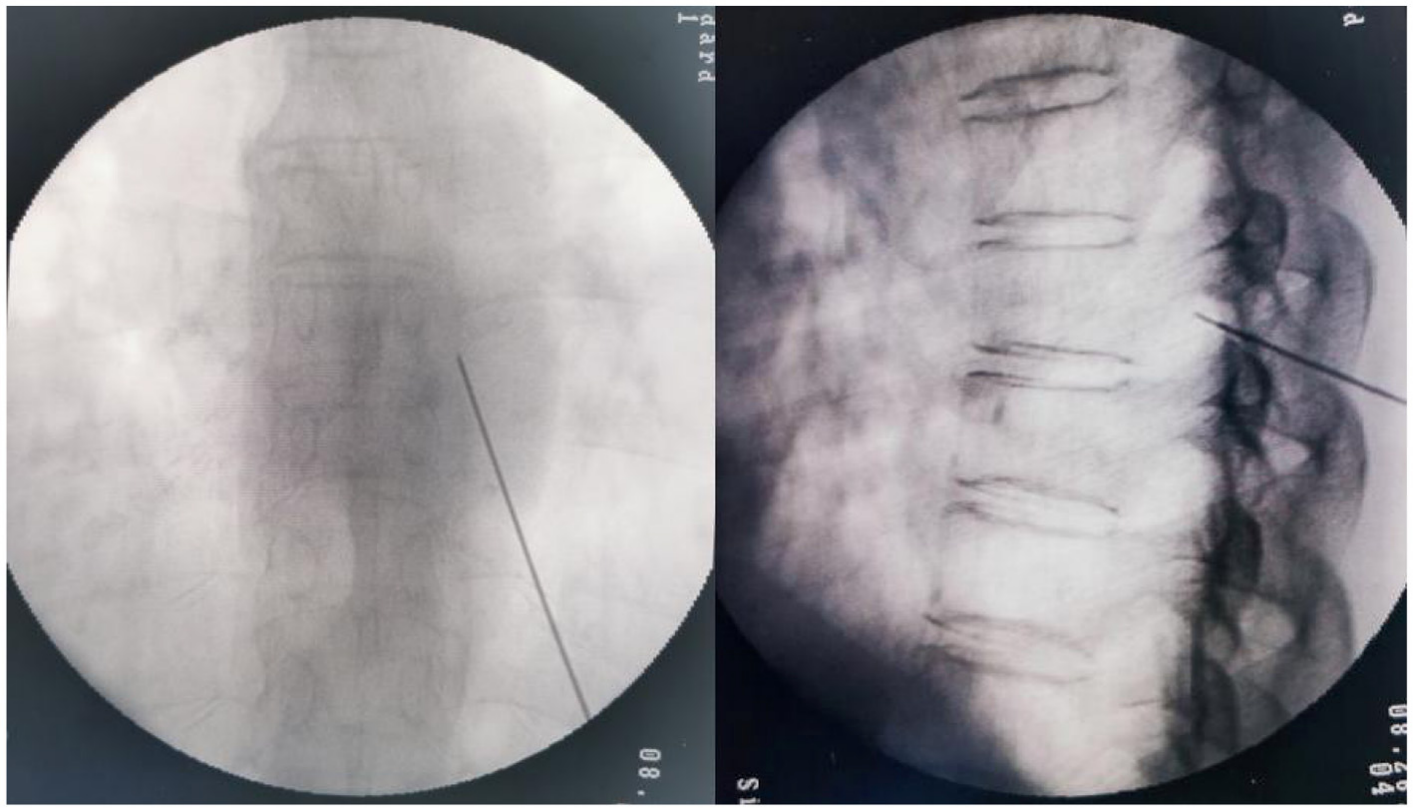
Puncture of the thoracic DRG under the guidance of an X-ray. The left is the anteroposterior view, and the right is the lateral view.
